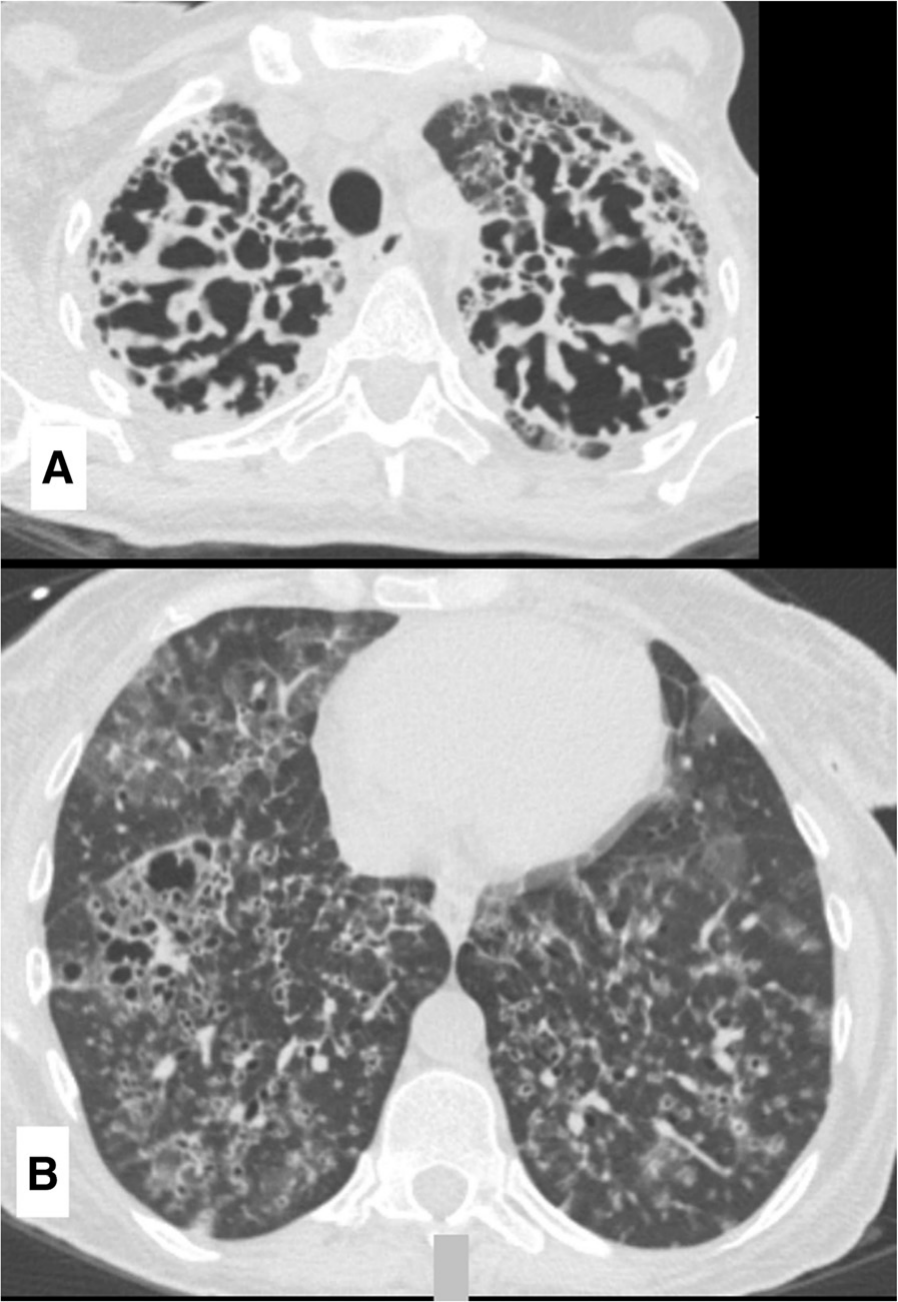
Computed tomographic scan of the patient’s chest at time of admission. (A) This image shows bilateral interstitial thickening and airspace destruction at the upper lung zones. (B) Ground-glass and cavitary opacities can be observed in the lower lung zones.
